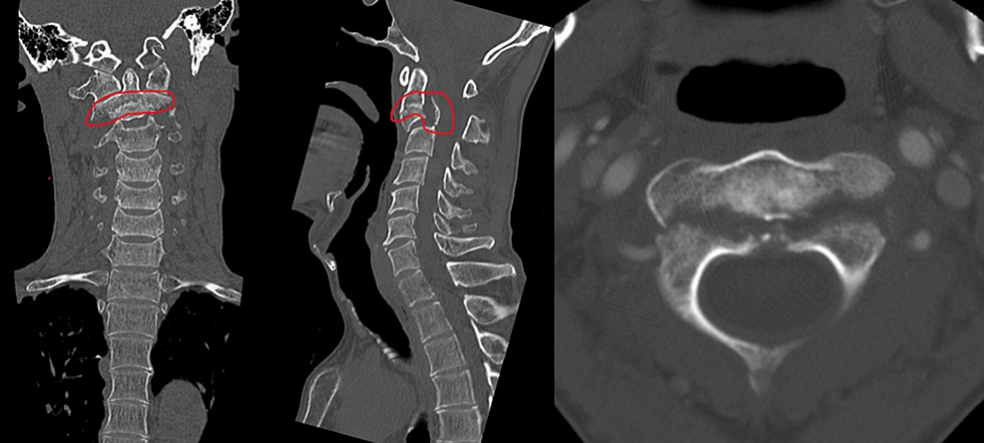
Plain CT images Plain CT showed fractures in the vertebral bodies as well as the intervertebral joints. Therefore, we determined that this patient required fixation, including C1.
